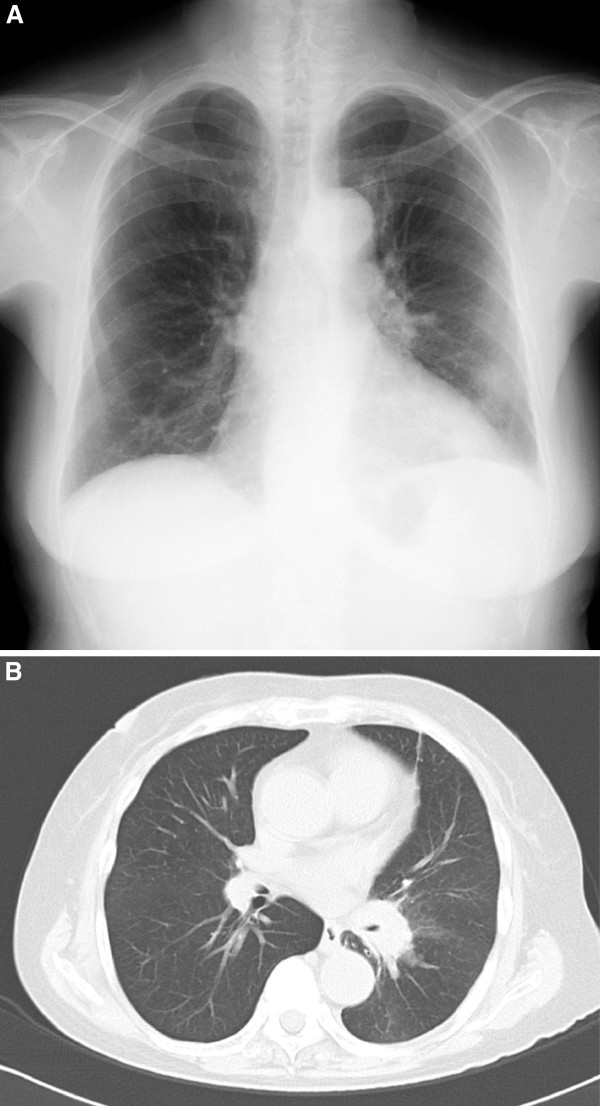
Chest imaging findings. (A) Posterior anterior view of the chest roentgenogram showing the left hilar and para-aortic tumor-like shadows. (B) Chest computed tomography (CT) imaging showing the tumor-like shadow surrounding the left lower bronchus.
